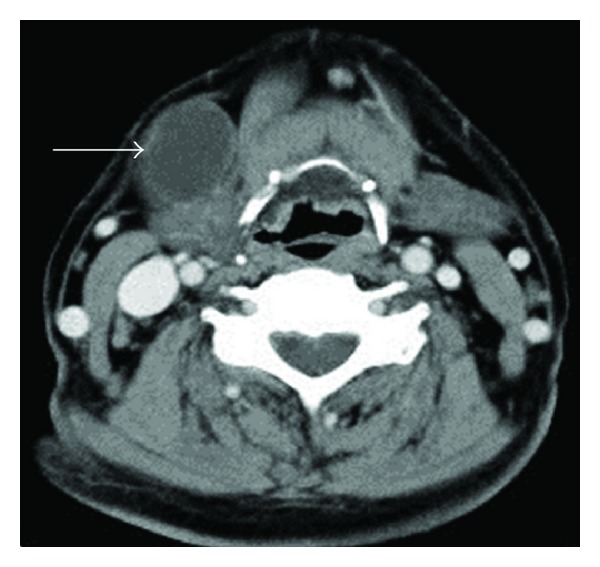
Contrast-enhanced CT (axial view). The cystic lesion was located anterior to the submandibular gland under the platysma and the margin of the mass was enhanced, suggesting a cystic lesion (arrow).
